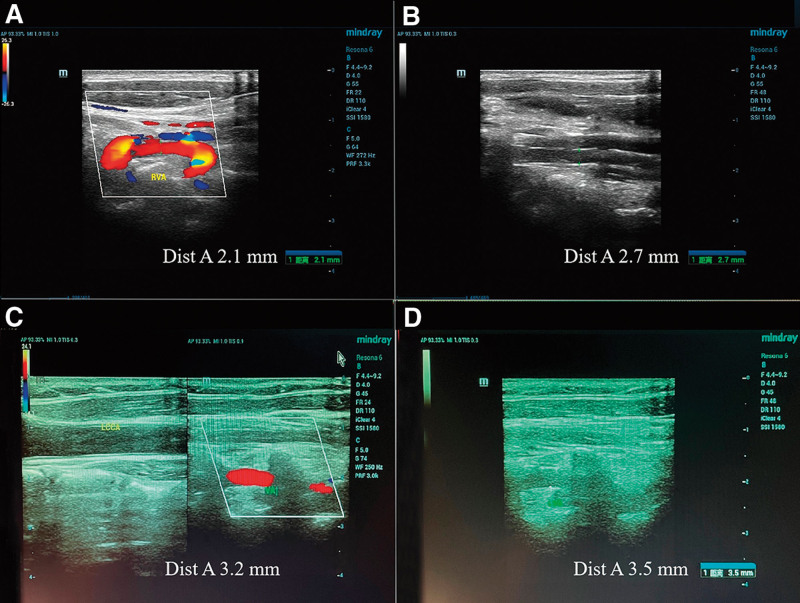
Carotid and vertebral artery ultrasound of 2 cases before and after intervention. Right vertebral artery (V2 segment) luminal diameter was (A) 2.1 mm before, and (B) 2.7 mm after intervention in Case 1. The same outcomes of left vertebral artery in Case 2 were (C) 3.2 mm before, and (D) 3.5 mm after intervention. Dist A = luminal diameter of vertebral artery.
